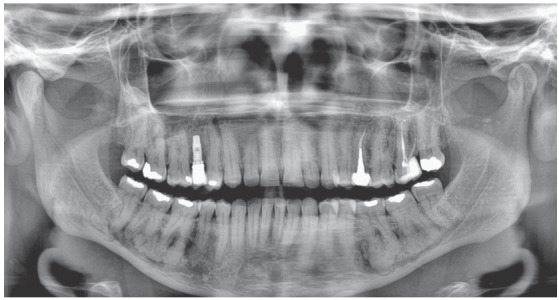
Panoramic radiograph of same clinical case with florid cemento-osseous dysplasia seen for evaluation of possible orthodontic treatment. Mandibular lesions are mixed, some radiopaque, some radiolucent, and are confused with roots of molar teeth, as they are randomly distributed in posterior region. Lesions have a cotton-wool appearance (or “flower beds”). Maxilla was not affected; implant is normal and functioning.
